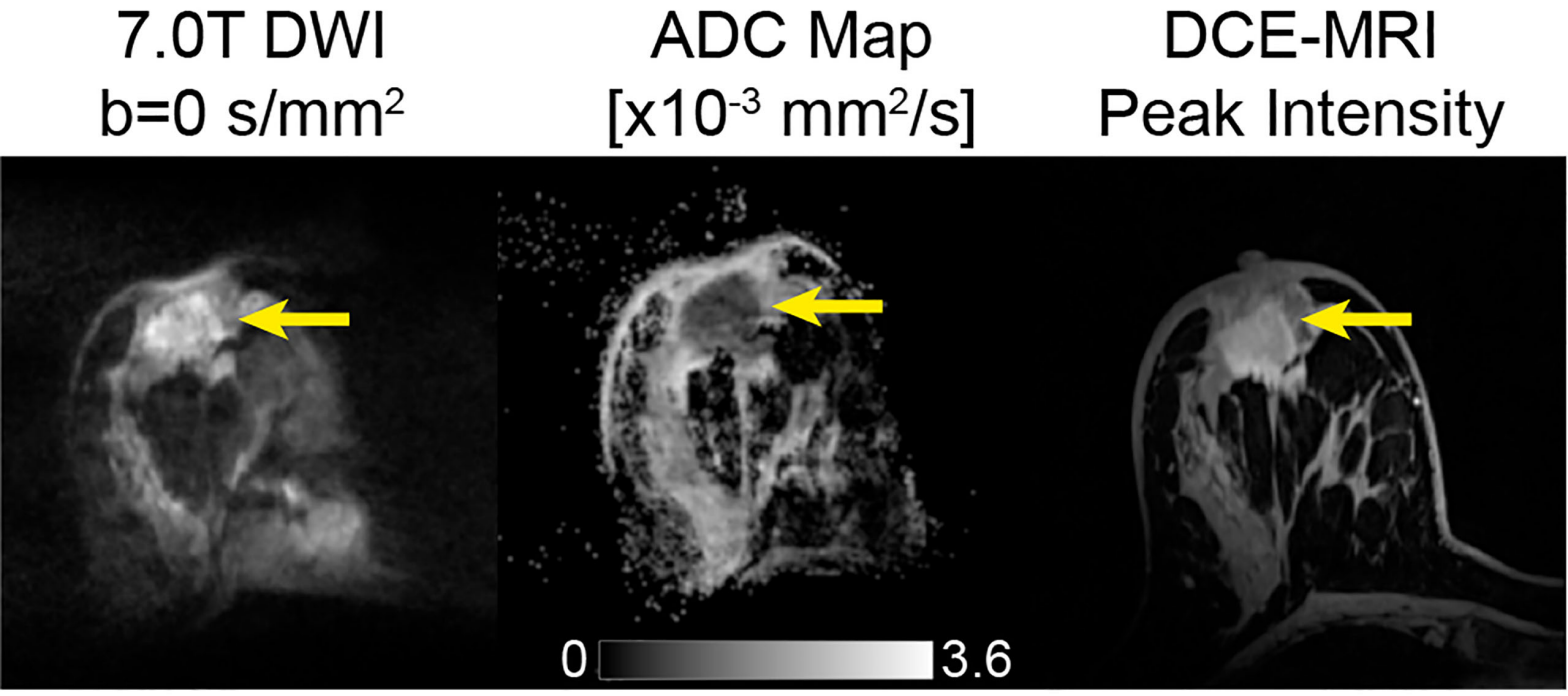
39-year-old female with invasive ductal carcinoma, imaged at 7T using a dedicated four-channel double-tuned ^31^P/^1^H breast coil. Images shown are b=0 s/mm^2^, corresponding ADC map, and DCE-MRI peak intensity subtraction. The mean ADC of the tumor shown is 0.71 ± 0.18 × 10^–3^ mm^2^/s. For DWI, a transverse DWI read-out segmented echo-planar imaging sequence was used. For DCE, patients were intravenously injected with a single dose of contrast agent (gadoterate meglumine) as a bolus, followed by a 20-mL saline flush after three of the 18 repetitions of the time-resolved angiographic imaging with stochastic trajectories sequence (26). (Courtesy of Katja Pinker-Domenig, MD, PhD, Department of Radiology, Memorial Sloan Kettering Cancer Center, New York, New York, USA).
